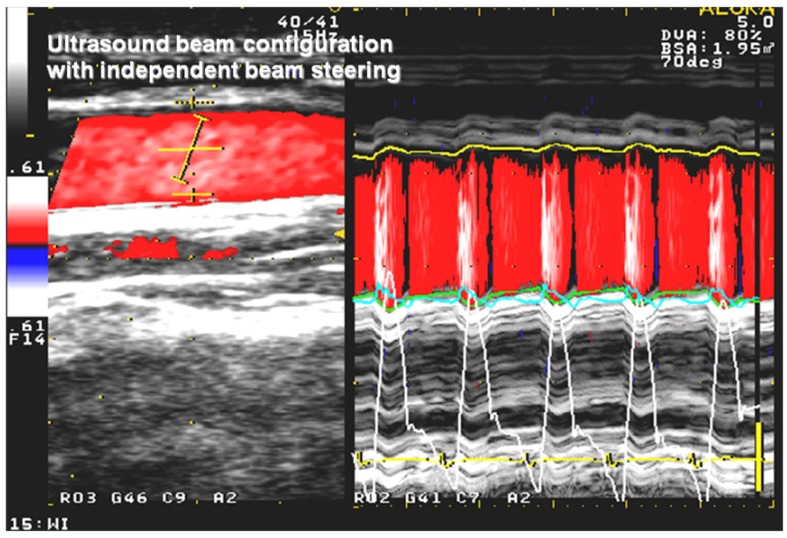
Echocardiogram of carotid artery with color flow and echo tracking beam localization (left side) and the diameter change waveform as the difference between posterior and anterior wall displacement (right side) assessed on the basis of echo tracking of the carotid artery calculated in real time (white curve for anterior wall, green for posterior wall) The white pressure-like configured curve corresponds closely to momentum of blood pressure.
